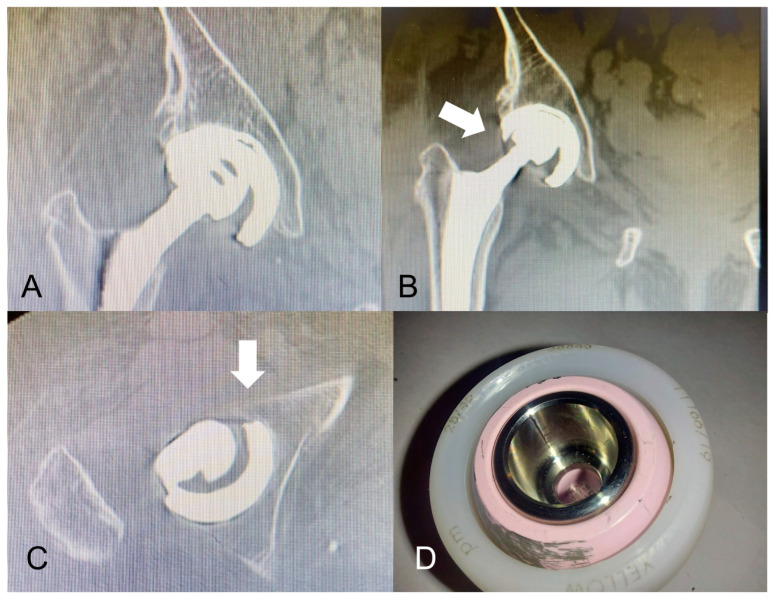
Early IPD of a modular DM construct occurred 11 months after revision surgery of a THA with a standard fixed polyethylene insert and 32 mm femoral head which previously failed for dislocation at a 19-month follow-up. CT scan (images (A–C)) was taken before re-revision. (A): CT scan image shows the eccentric position of the small femoral head with respect to the acetabular shell. It is well recognized the modular adapter between the ceramic head and the femoral stem morse taper. (B): the modular CoCr liner appears to be malseating into the titanium acetabular shell: the superior-lateral margin of the metal liner (white arrow) is more prominent from the acetabular shell equatorial rim than its medial margin. Another radiographic sign of modular liner malseating is the eccentric void space between the liner and shell. (C): white arrow shows how the acetabular cup was placed with excessive anteversion and modular CoCr liner malseating. (D): wear signs on the retrieved ceramic head surface due to its articulation against the modular CoCr liner edge.
